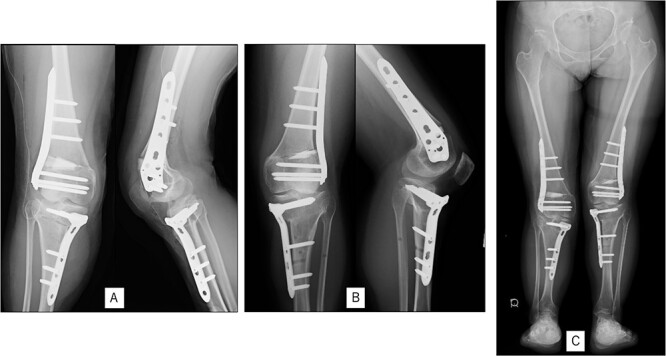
Postoperative radiography: (A) immediate postoperative radiograph of the right knee, (B) immediate postoperative radiograph of the left knee, (C) the entire lower limbs at 2 months after left knee surgery. Mild valgus deformity of the lower limbs persisted.
